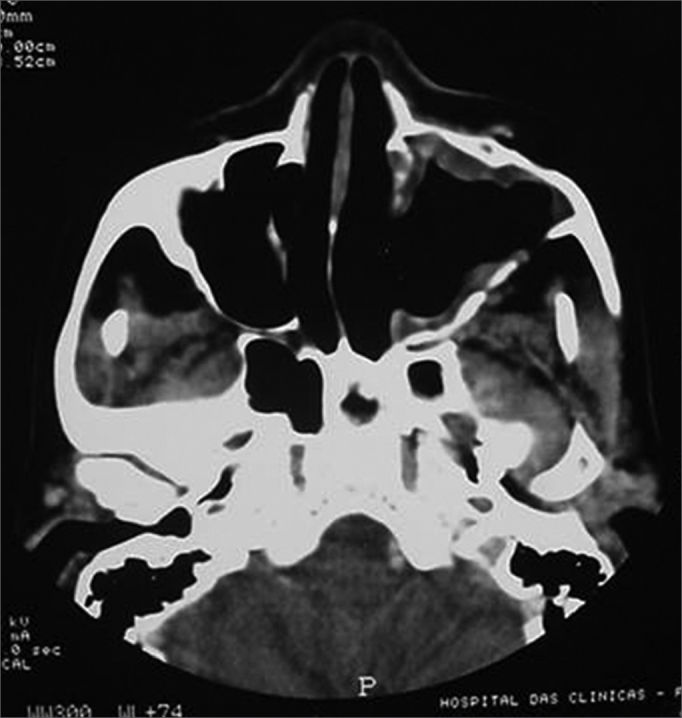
CT axial slice, soft tissue window.
